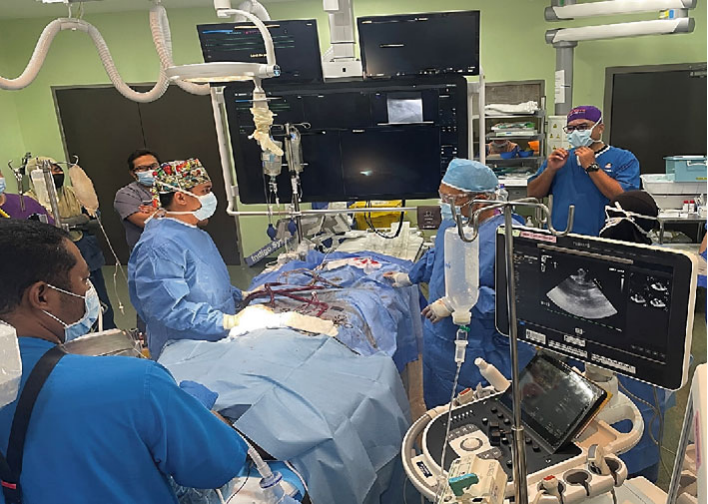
Cardiac anesthesiologist performing transesophageal echocardiography (TOE) to confirm the placement of ECMO cannulas.
